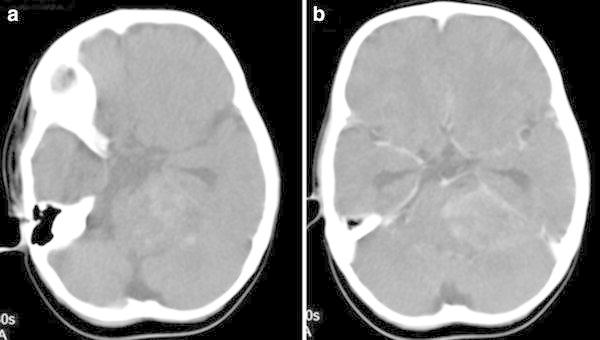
a, b On CT images without contrast, isodense with the cerebellar parenchyma in the left CPA, amorphous calcifications including an extra-axial, dural-based mass lesion 4 × 3 cm in size, with heterogenous contrast after the application of IV contrast dye. Time there is concomitant calcification, bleeding and oedema of the surrounding
